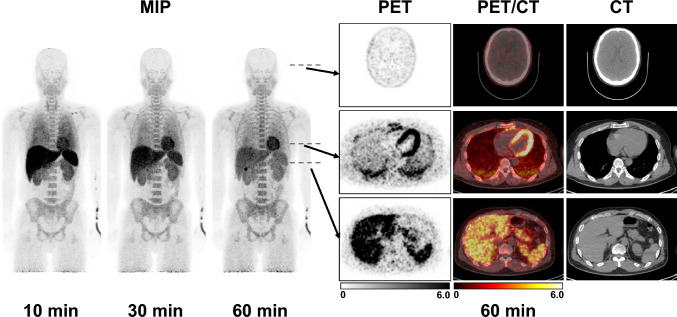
[^18^F]FEDAC-PET/CT Imaging in Normal Volunteer. Representative images (MIP images after 10 min, 30 min, and 60 min after injection and axial images of the head, chest, and upper abdomen.). The first 10-min image shows strong accumulation in the lungs, heart, liver, spleen, kidney, and bone marrow. Subsequently, the accumulation in the liver diminishes with time, and the radiotracer migration is seen from the bile duct to the gastrointestinal tract
